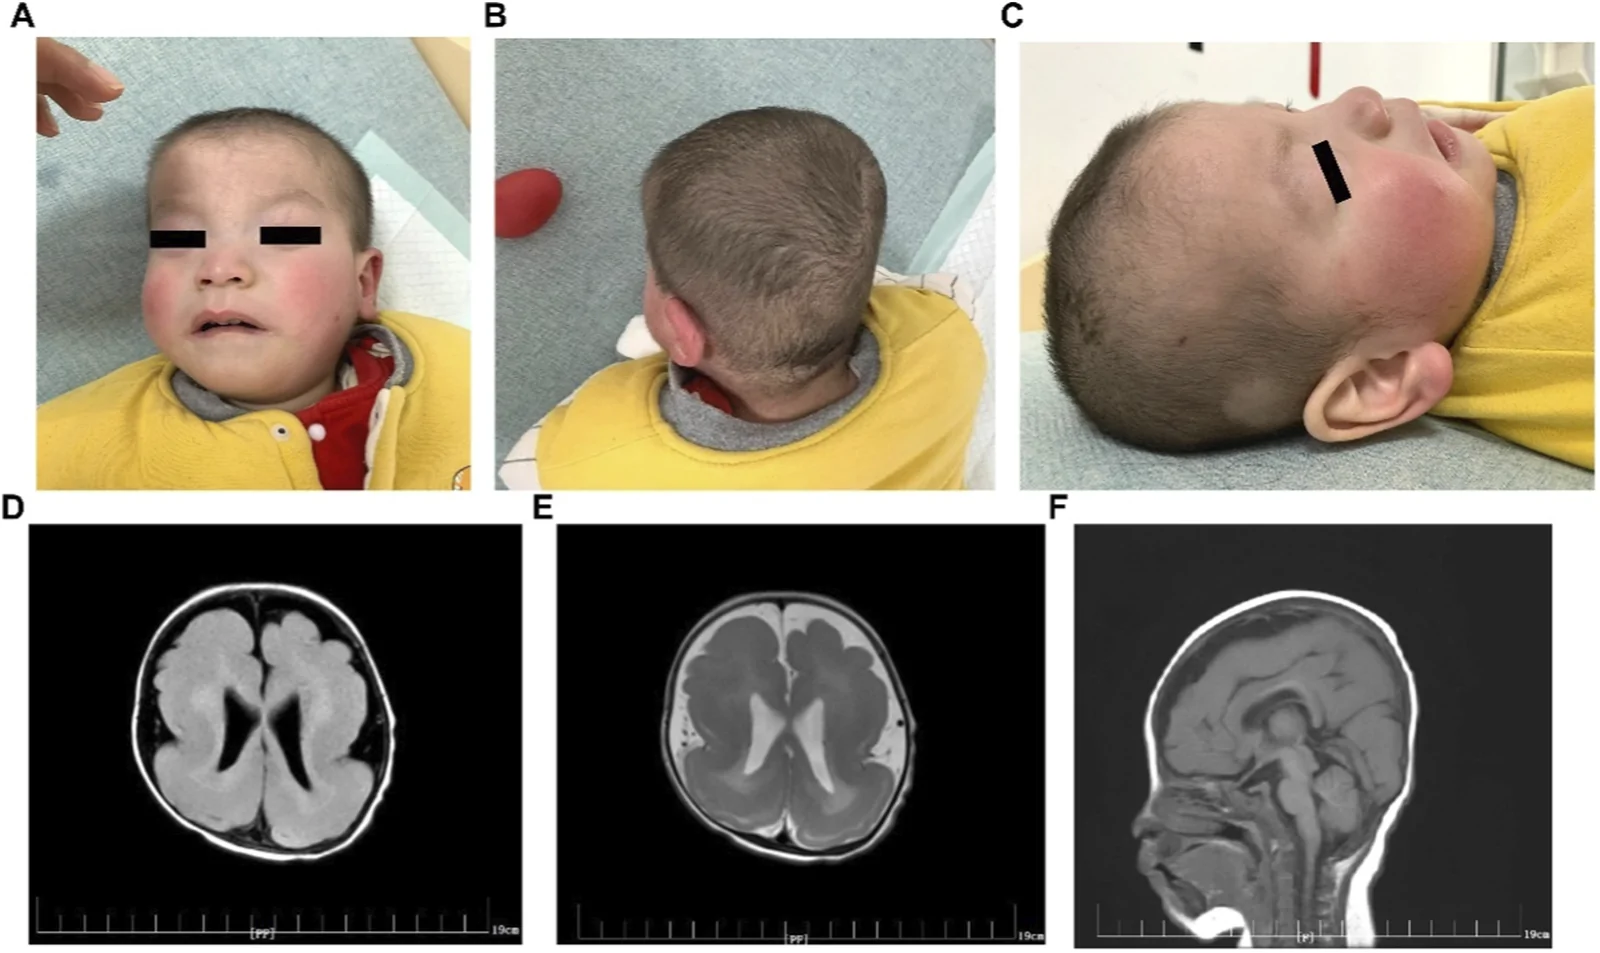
Craniofacial features and brain MRI imaging findings of the proband. (A–C) Photographs of the proband showing typical facial features of ILS, including microcephaly, prominent forehead, midface hypoplasia, and characteristic dysmorphic facies. (D–F) Cranial MRI scans showing abnormal neuronal migration. Axial views demonstrate widened gyri, shallow sulci, and a simplified cerebral cortex. Sagittal view reveals unclear morphological definition of the rostrum and genu of the corpus callosum, suggestive of hypoplasia or delayed myelination.
